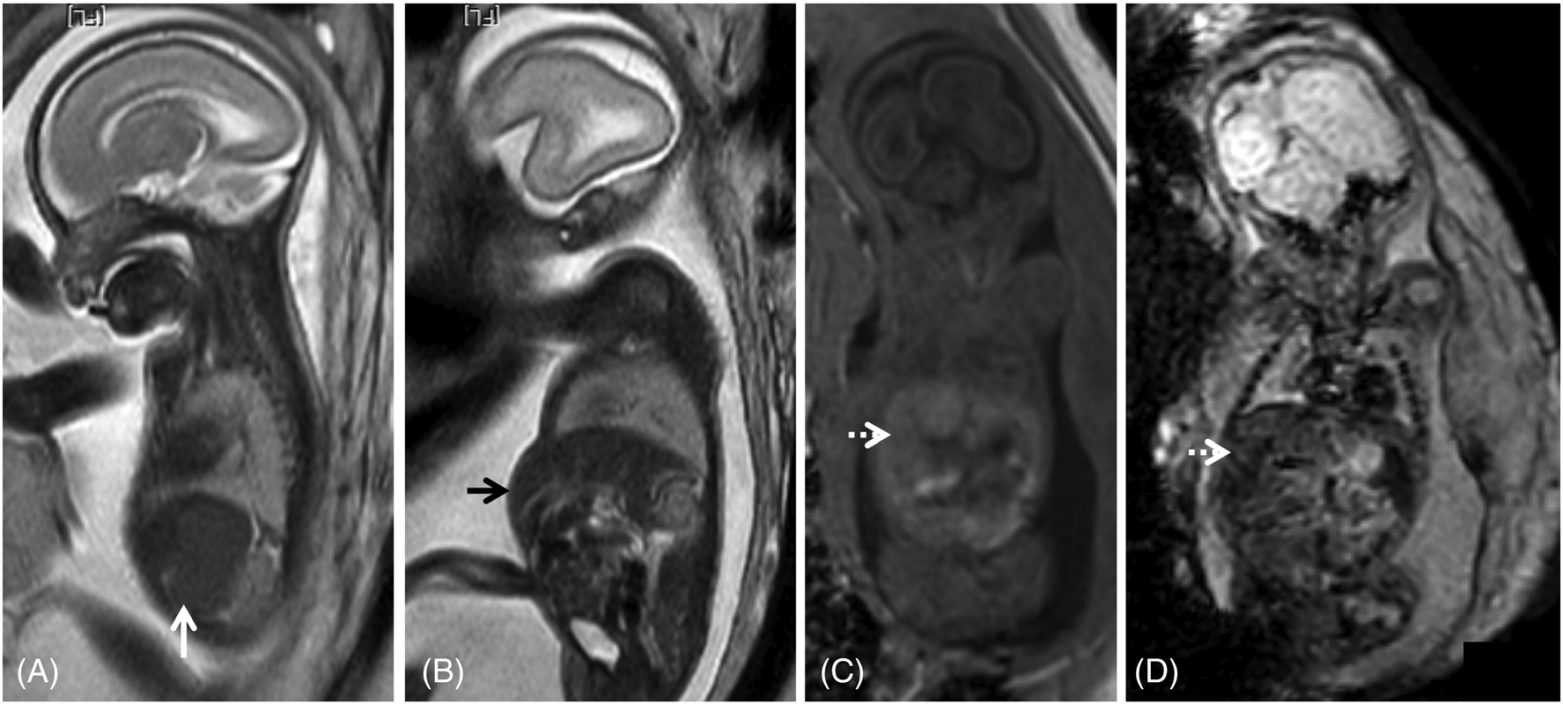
Fetal magnetic resonance imaging of a cytomegalovirus (CMV) positive fetus at 24 gestational weeks. T2WI depict splenomegaly (A, white arrow) and hepatomegaly (B, black arrow), protruding in the anterior abdominal wall. There is associated abnormal liver signal: isointense on T1WI (C, dashed white arrow) and on echo planar images (D, dashed white arrow), which cannot be identified on T2WI. These findings are often found in fetal infections and may help guide the diagnosis when brain anomalies are found, in the absence of a definite diagnosis of CMV infection
